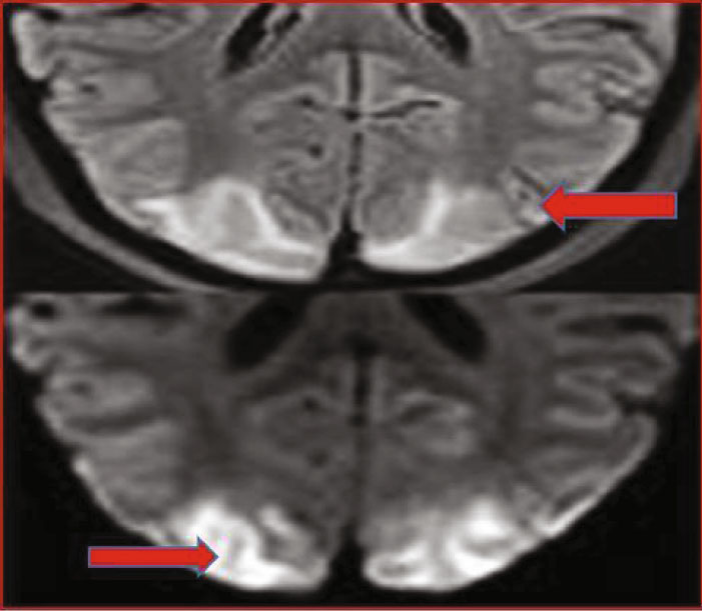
MRI of the brain showing posterior reversible encephalopathy syndrome.
